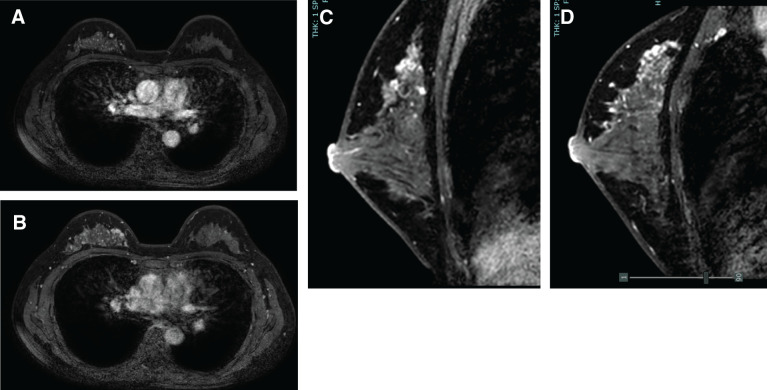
Contrast-enhanced breast MRI demonstrated a 5-mm enhancing nodule, and linear and branching non-mass enhancement with high signal intensity on T1WI from the upper inner quadrant to the upper outer quadrant of the right breast (A, B). On sagittal images, non-mass enhancement was observed in both the upper inner quadrant to the upper outer quadrant of the right breast (C, D). T1WI, T1-weighted image
